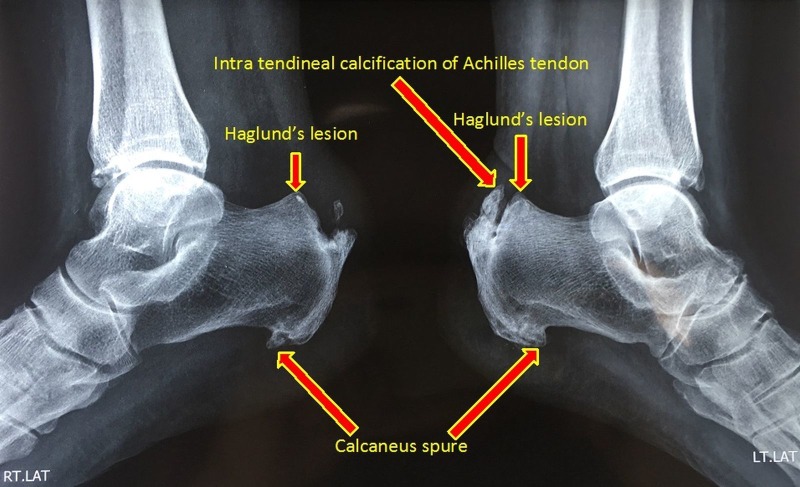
Lateral radiograph of ankles showing bony enlargement at the posterosuperior aspect of both calcaneum
